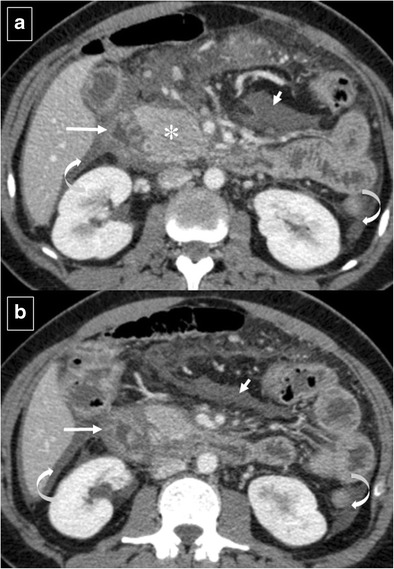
Acute exsudative pancreatitis and duodenal edema in a 50-year-old man. Contrast-enhanced CT shows an enlarged pancreatic head (*) with stranding of the peripancreatic fat and peripancreatic fluid (short arrows). The wall of the duodenum is thickened, and limited mural enhancement is seen secondary to edema (arrows). Also note the pathologic amount of fluid in the upper abdomen (curved arrows)
